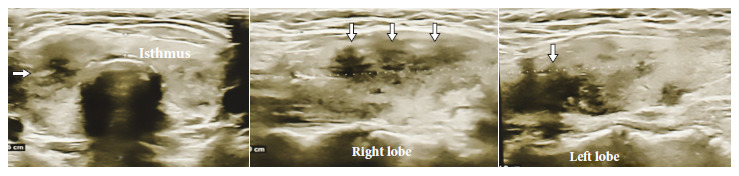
Case 1. Thyroid ultrasound showing poorly defined hypoechogenic areas (white arrows) compatible with subacute thyroiditis.
